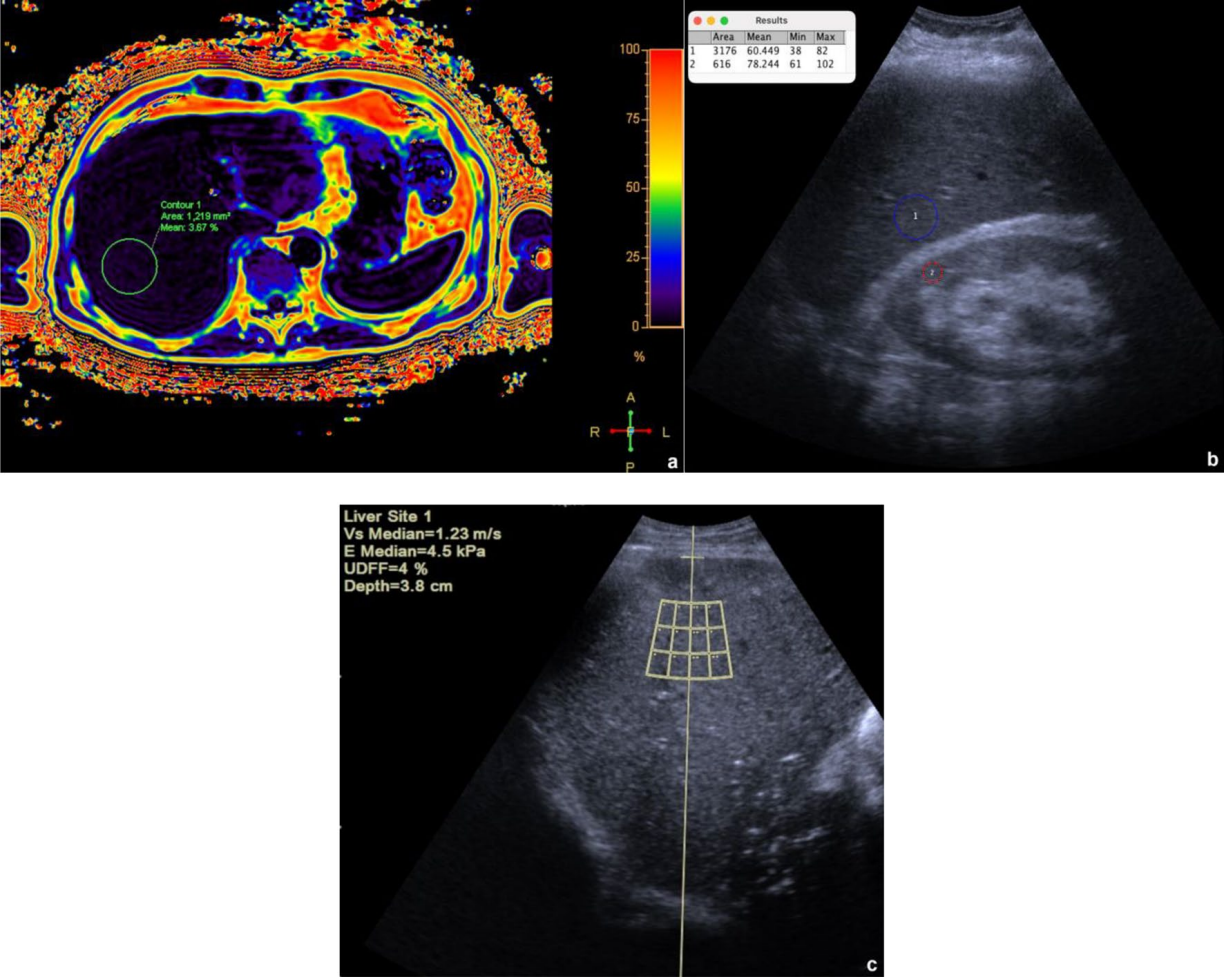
Quantification of liver fat content in a 58-year-old woman without steatosis. a Fat fraction map: a circular ROI is visible in liver segment 7; the overall median MRI-PDFF value was 4%. b B-mode US and quantification of the hepatorenal index: liver echogenicity is comparable to the right kidney cortex; the ratio of the mean brightness of liver (1) and kidney (2) ROIs was 0.77; c UDFF measurement: a ROI box is visible in liver segment 7; the overall median UDFF value was 4%
